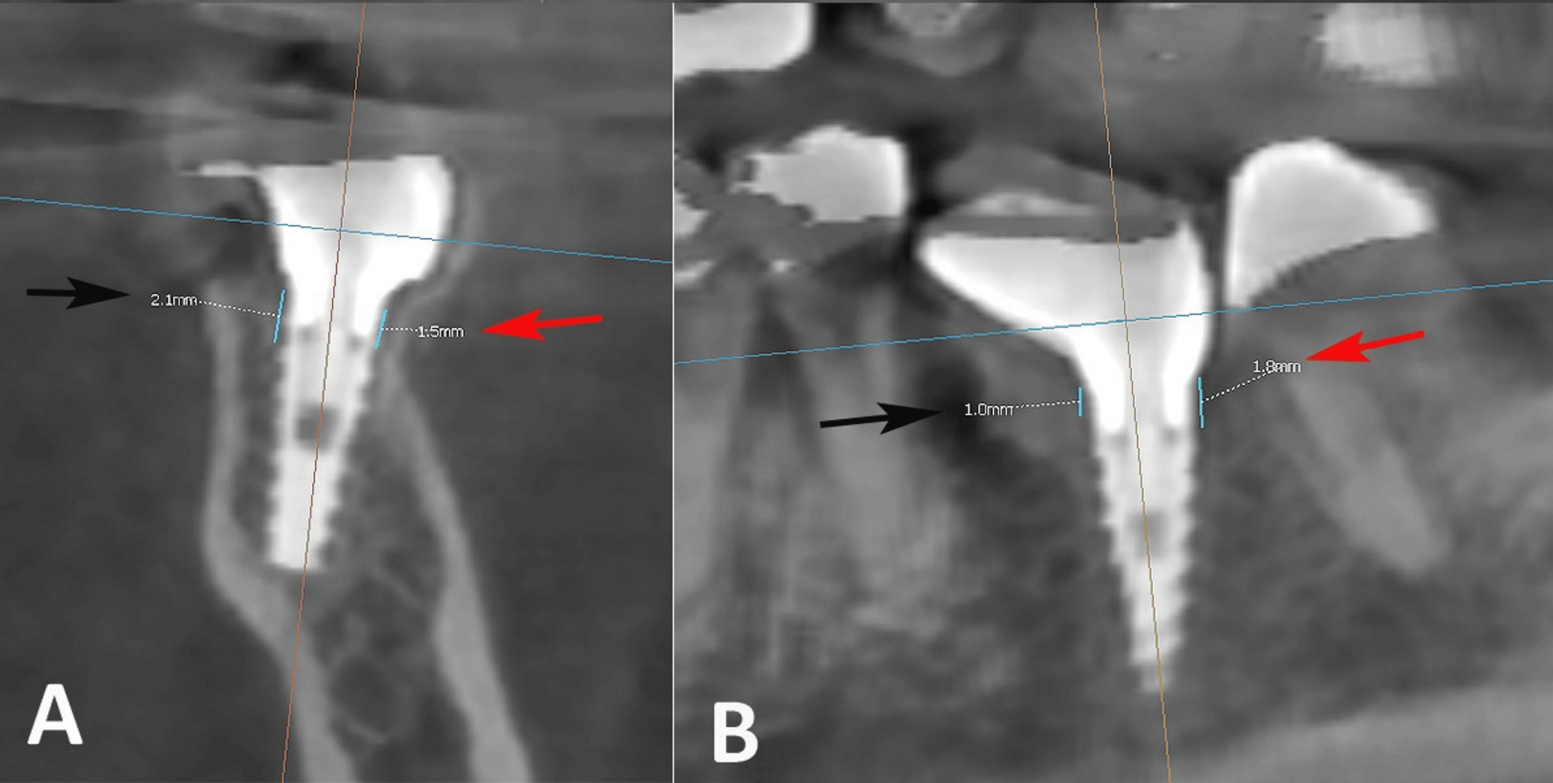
(A) CBCT coronal view of a dental implant demonstrating crestal bone loss on the buccal (red arrow) and lingual (black arrow) surfaces, (B) CBCT sagittal view showing crestal bone loss on the mesial (black arrow) and distal (red arrow) surfaces. Crestal bone loss was measured from the implant platform to the nearest apical bone–implant contact. Original CBCT images of the patient, used with the patient's permission. CBCT: Cone-beam computed tomography
